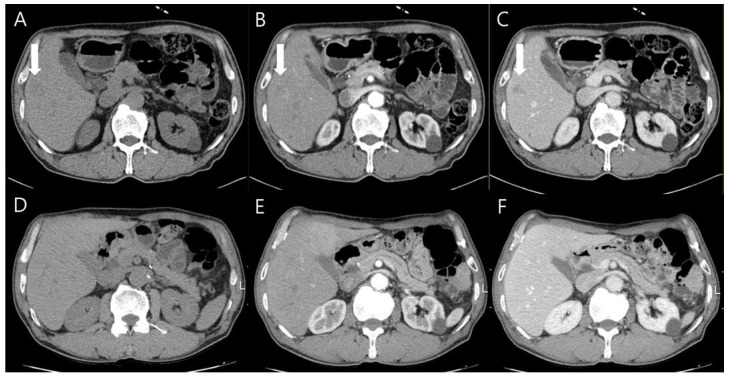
Abdominal CT images showed the solitary 2 cm ill-defined nodule in segment 5/6 of the liver ((A–C), arrows). It was presented as an ill-defined low-attenuation nodule in the pre-contrast phase (A), poorly delineated in the arterial phase (B), and also visible in the portal phase (C). After 6 months, the nodule was invisible in all phases (D–F).
